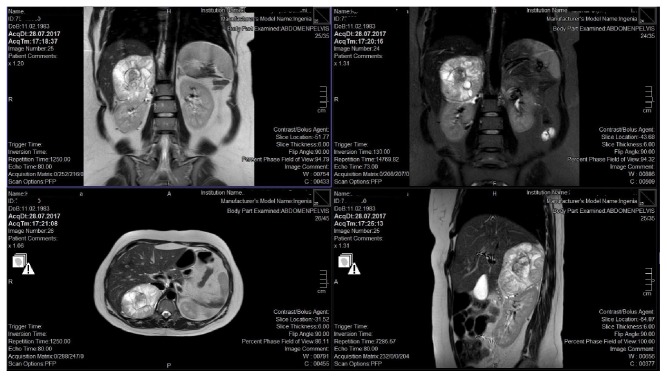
MRI examination showing a 11×7.5 cm right suprarenal hypervascular mass with mixed signal intensity in T2-weighted images.
